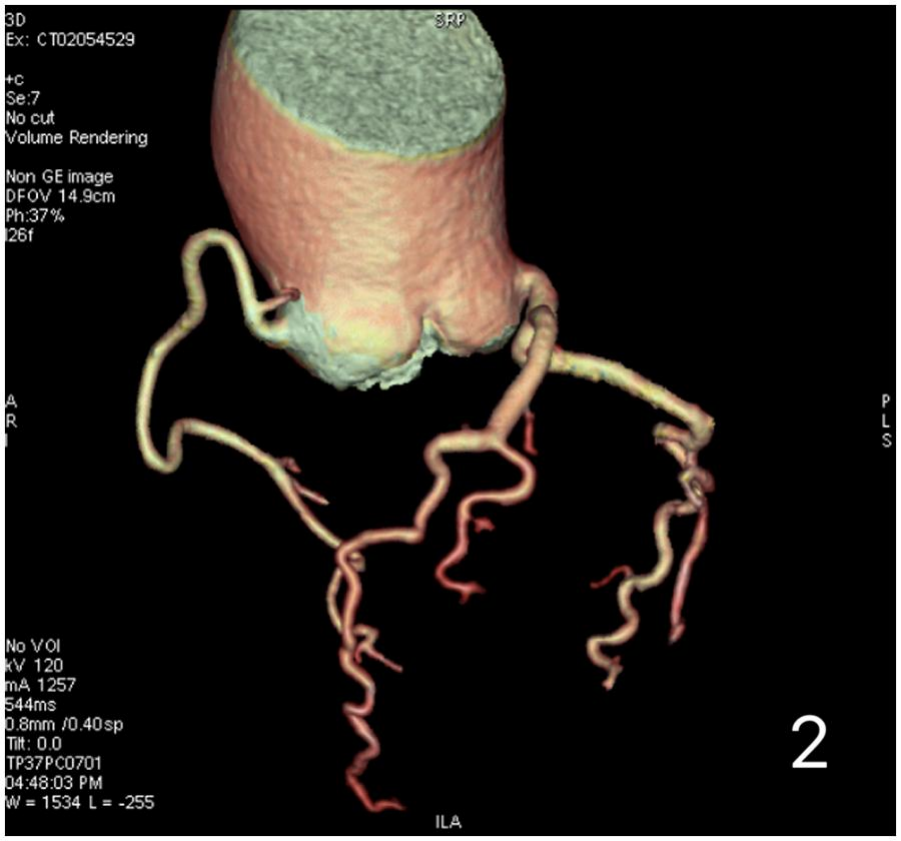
Preoperative coronary computed tomography angiography (CTA) of the patient demonstrates no significant coronary artery stenosis.
